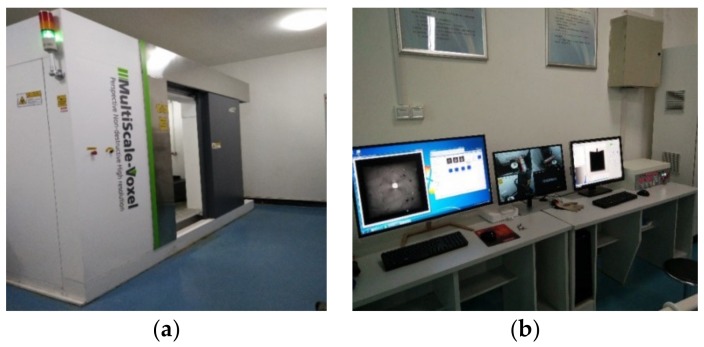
The Industrial Computed Tomography (ICT) equipment. (a) The ICT Scanning Equipment; (b) The ICT Operating Equipment.
